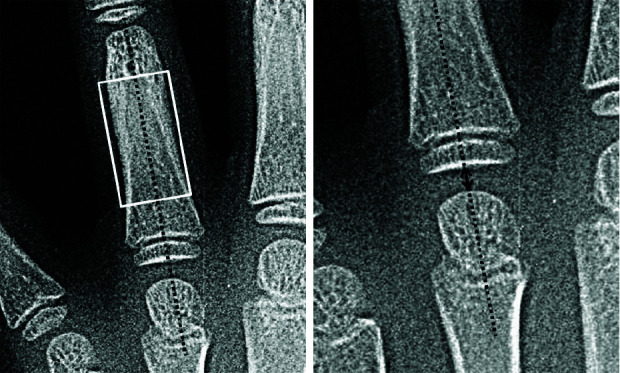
4^th^ metacarpophalangeal joint width in hand radiographs
